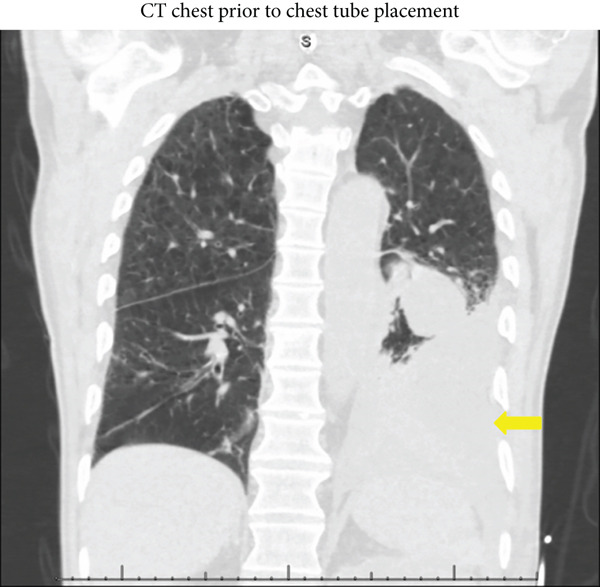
A CT chest in the coronal view taken prior to chest tube placement displaying a large loculated collection (yellow arrow) throughout the left lung base.
